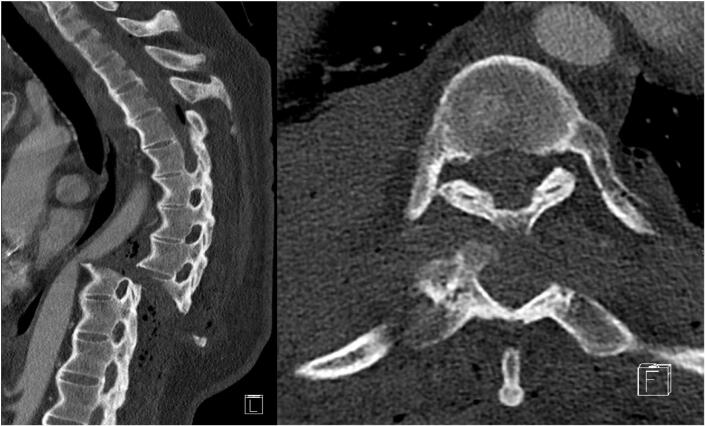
CT imaging at admission (left: sagittal, right: axial), C Type fracture with anterior fracture displacement and proximity to the aorta.
